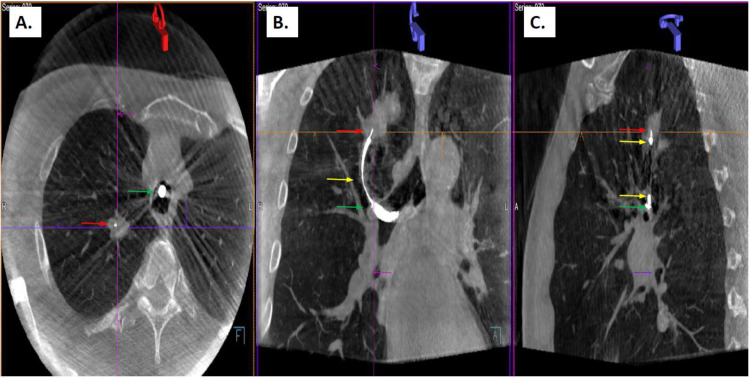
Representative Cone Beam Computed Tomography imaging showing interaction between biopsy tool (red arrow head, 21-gauge Arcpoint^TM^ needle (Medtronic, Plymouth, Minnesota)) placed through extended working channel (yellow arrow head) of electromagnetic navigation system (superDimension version 7.1 (Medtronic, Plymouth, Minnesota)) inside the flexible bronchoscope (green arrow head) and the target peripheral pulmonary nodule in the right upper lobe (A) axial reconstruction, (B) coronal reconstruction, (C) sagittal reconstruction) (purple arrows and lines are part of the Siemens software analysis program).
